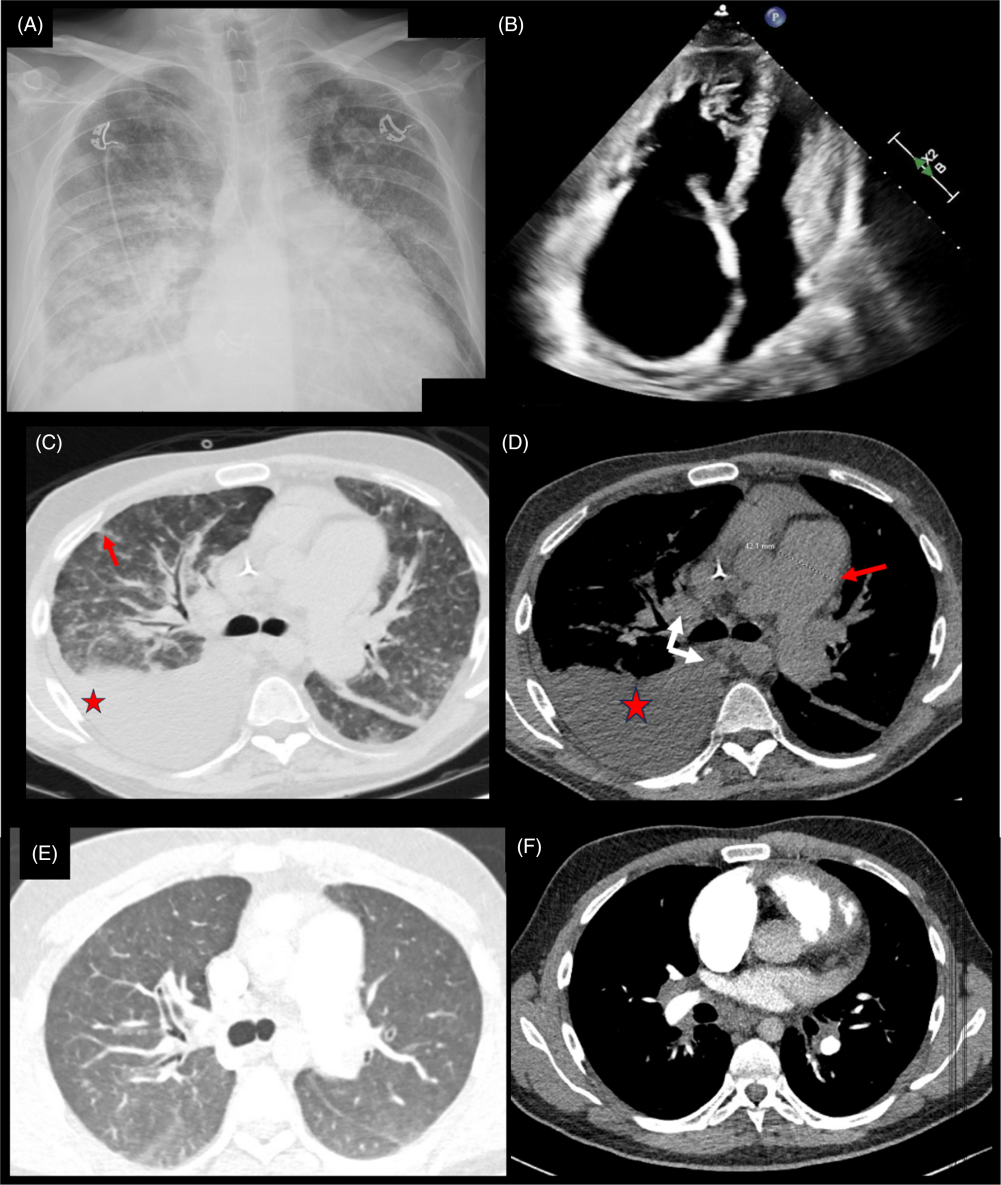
Chest x‐ray, transthoracic echocardiogram, and CT imaging from patient on admission for refractory right ventricular failure and during period of clinical stability. (A) Chest x‐ray on admission for volume overload and RV failure showing diffuse interstitial and alveolar opacities and cardiomegaly. (B) Apical 4‐chamber view on echocardiography showing flattened septum consistent with RV volume and pressure overload, and severe right atrial dilation. (C, D) High‐resolution CT Chest prior to lung transplantation, with (C) lung windows showing ground‐glass opacities, presence of septal thickening (red arrow), and (D) mediastinal windows showing severely enlarged pulmonary artery (red arrow), mediastinal lymphadenopathy (white arrows), and large right‐sided pleural effusion (red star). E/F: On CT Chest from six years prior to admission, patient had revealed (E) diffuse heterogeneous ground glass opacities, centrilobular nodularity with evidence of septal thickening, evidence of pulmonary venous extension to the periphery of the lung fields with tortuosity, and (F) substantial hilar lymphadenopathy.
